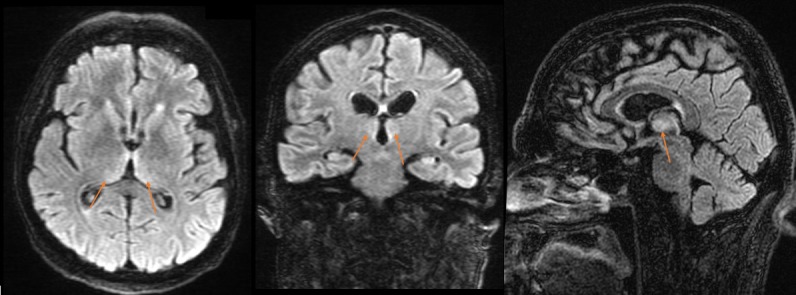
MRI head: axial, coronal, and sagittal FLAIR sequences, demonstrating periventricular hyperintensities at the medial thalami bilaterally, consistent with Wernicke’s encephalopathy. Orange arrows identifing areas of abnormality.
